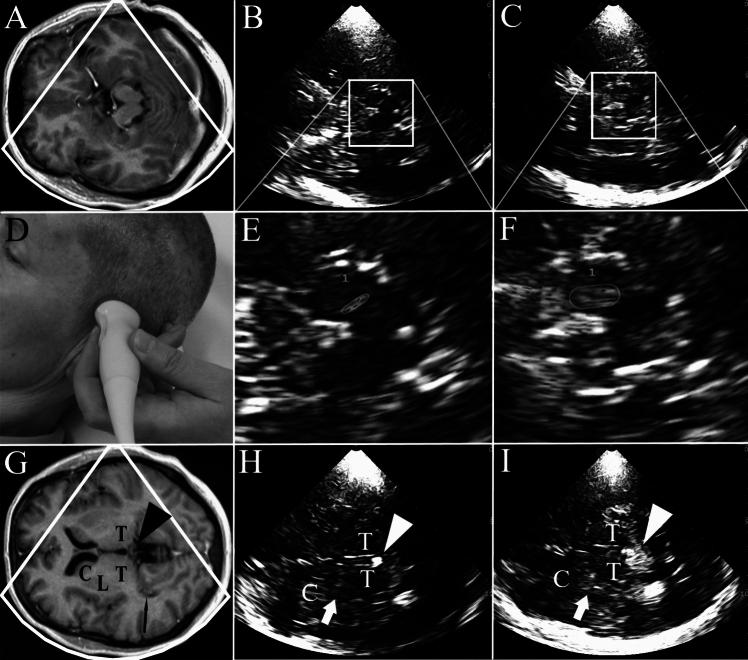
Transcranial sonography (TCS) in the diagnosis of Parkinson’s disease (PD). A MRI of midbrain axial transection corresponding to the TCS images shown in (B) and (C). B Axial TCS scan at midbrain level in an individual with normal aspect of substantia nigra (small echogenic area, SN–). This finding is typical for essential tremor and multiple system atrophy. C Axial TCS scan at midbrain level in an individual with enlarged echogenic size of substantia nigra (hyperechogenicity, SN +). This finding is typical for PD. D Position of the ultrasound transducer for diagnostic TCS in PD. E Zoomed TCS image of midbrain shown in (B). The echoic area of substantia nigra is traced for automated measurement. F Zoomed TCS image of midbrain shown in (C). The echoic area of substantia nigra is traced for automated measurement. G MRI of basal-ganglia axial transection corresponding to the TCS images shown in (H) and (I). *C* caudate nucleus, *L* lenticular nucleus, *T* thalamus, arrow head: pineal gland. H Axial TCS scan at basal-ganglia level in an individual with normal aspect of lenticular nucleus (weakly echogenic, LN−; arrow). This finding is typical for PD. I Axial TCS scan at basal-ganglia level in an individual with increased echogenicity of lenticular nucleus (LN + ; arrow). This finding is frequent in atypical Parkinsonian syndromes
